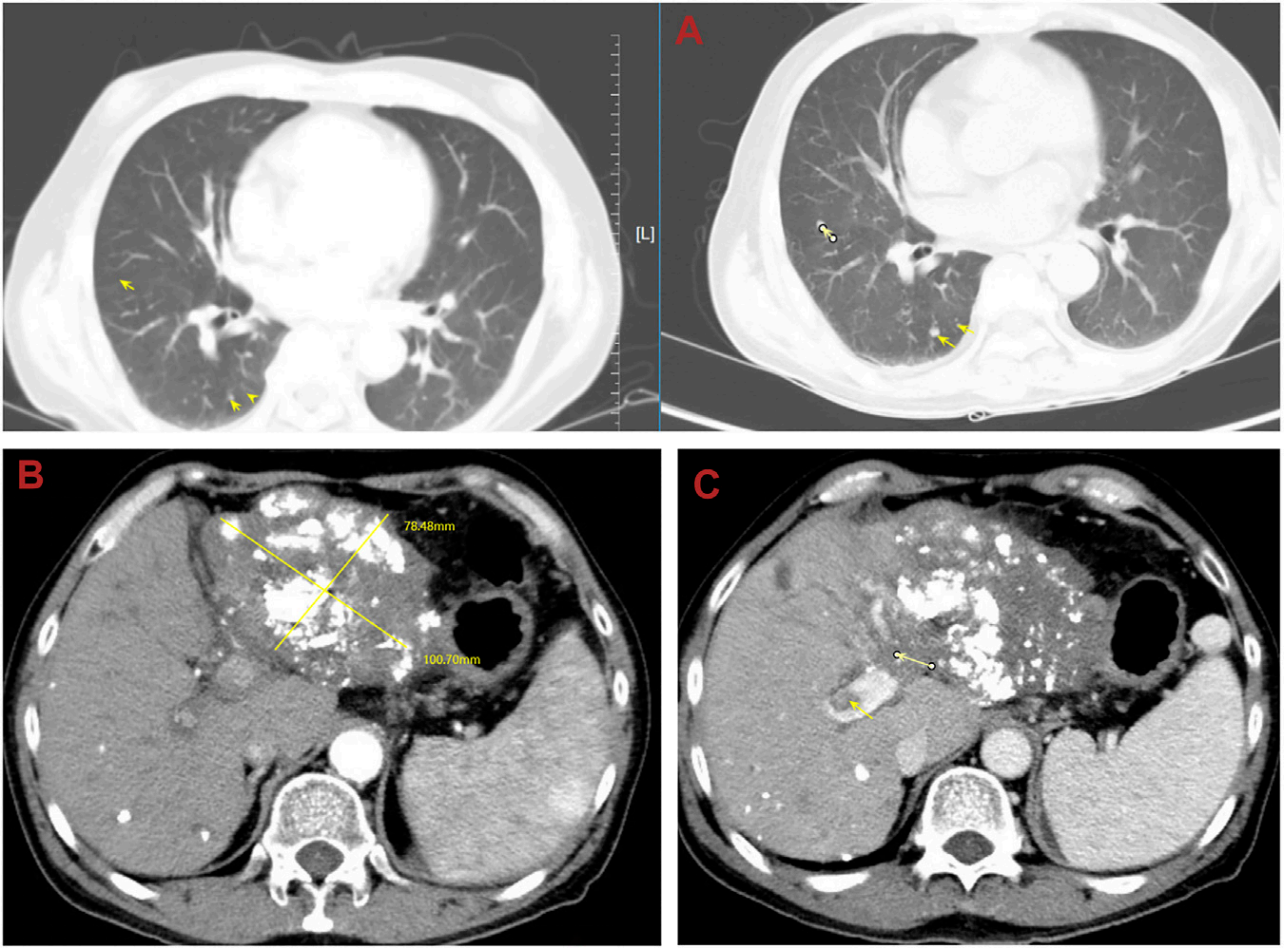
Abdominal computed tomography (CT) shows that multiple small nodules in both lungs were significantly reduced and shrunken and the tumor in the liver was significantly reduced. (A) The lung metastases was reduced and shrunken; (B) the tumor in the left lobe of the liver shrunk; (C) the tumor thrombosis in the left portal vein.
